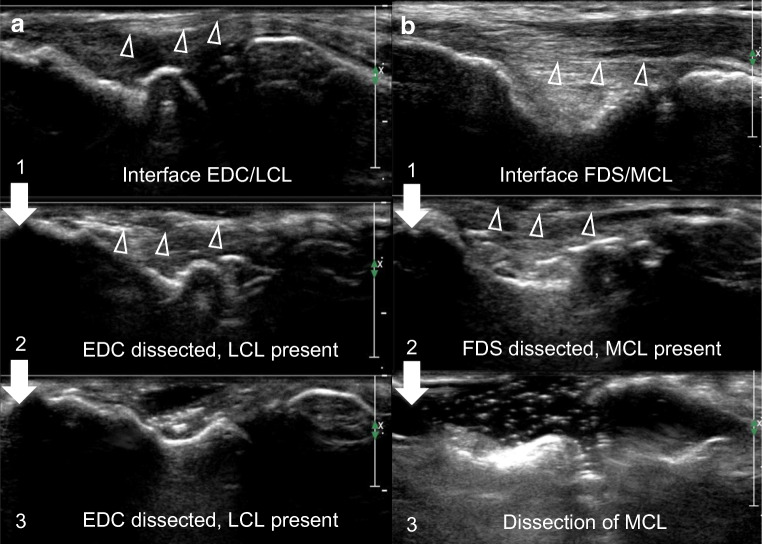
Progressive layer by layer dissection technique. A progressive layer by layer dissection technique is presented for the lateral (a) and medial (b) epicondyles. Each structure is identified at ultrasonography. The identified structure is then resected at dissection. The ultrasonography is repeated, with the confirmation of the correct identification of anatomical structures. In a, arrowheads point at the interface between the EDC and LCL. In b, arrows point at the interface between the FDS and the MCL. EDC: extensor digitorum communis, LCL: lateral collateral ligamentous complex, FDS: flexor digitorum superficialis, MCL: medial collateral ligamentous complex
